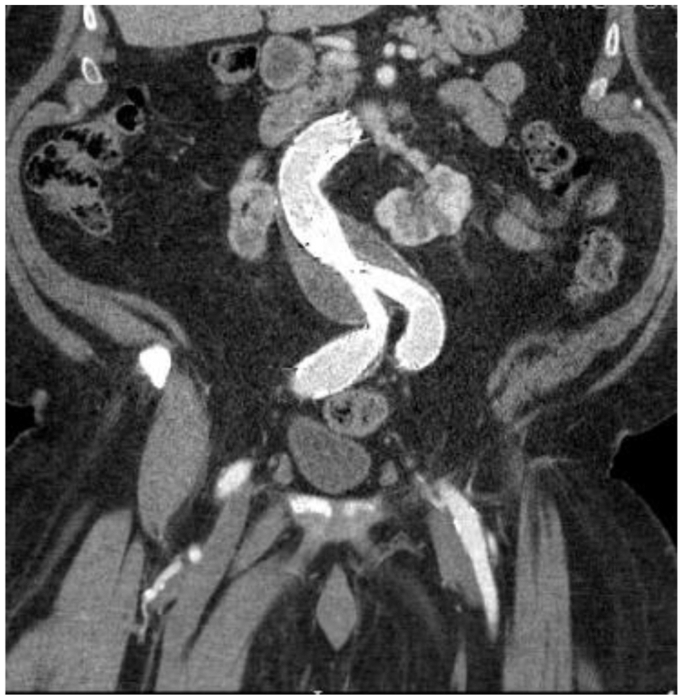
Coronal computed tomography (*CT*) imaging of patent endograft at 1-year surveillance appointment.
